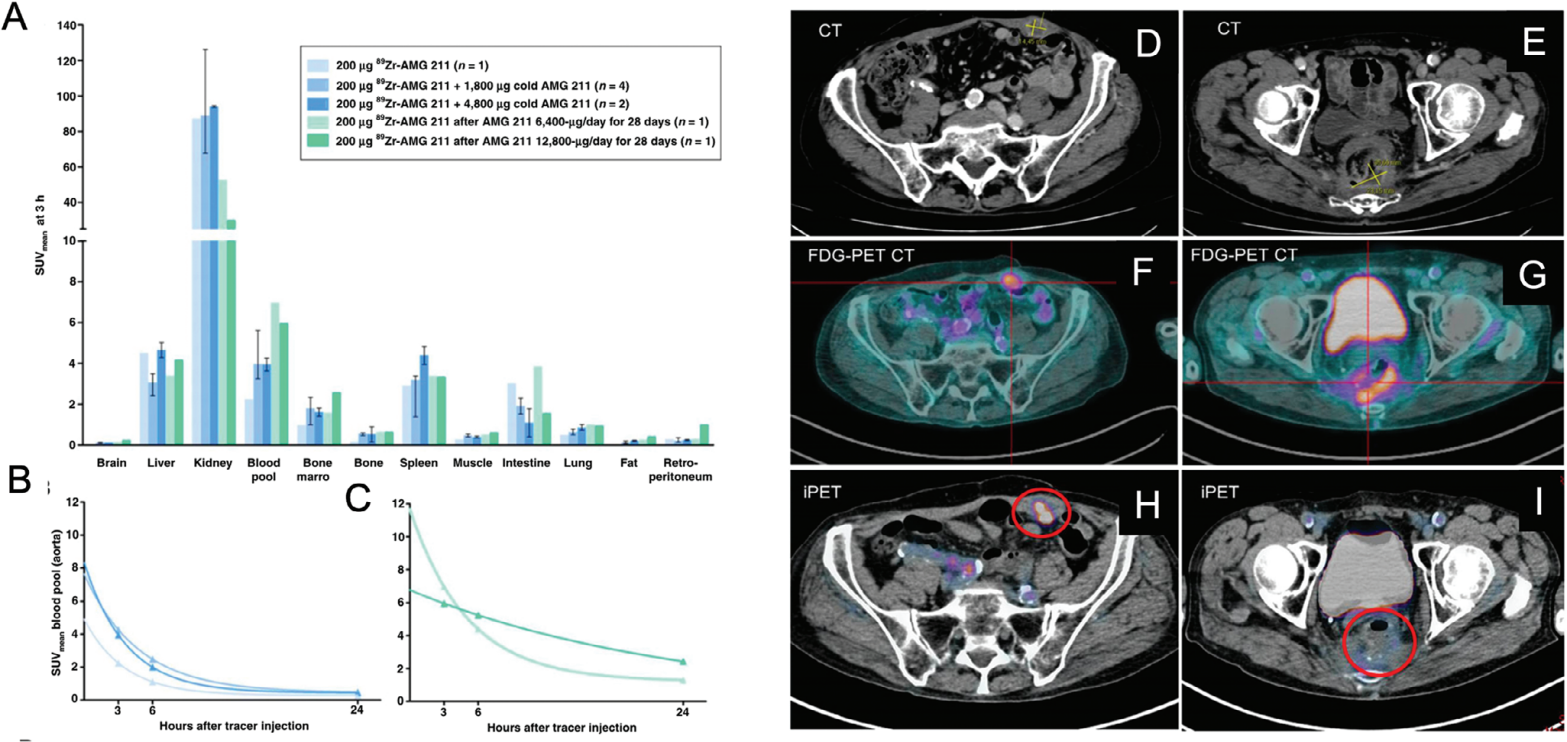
Biodistribution of ^89^Zr‐AMG 211. A) ^89^Zr‐AMG 211 healthy tissue biodistribution at 3 h post tracer administration for the different dosing cohorts used for imaging before (blue) and during (green) AMG 211 treatment. Data are shown as median SUVmean, error bars. B,C) Nonlinear regression curve showing mean SUVmean in the blood pool measured in the thoracic aorta per PET scan time B) before AMG 211 treatment and C) during AMG 211 treatment. Reproduced with permission.^[^
